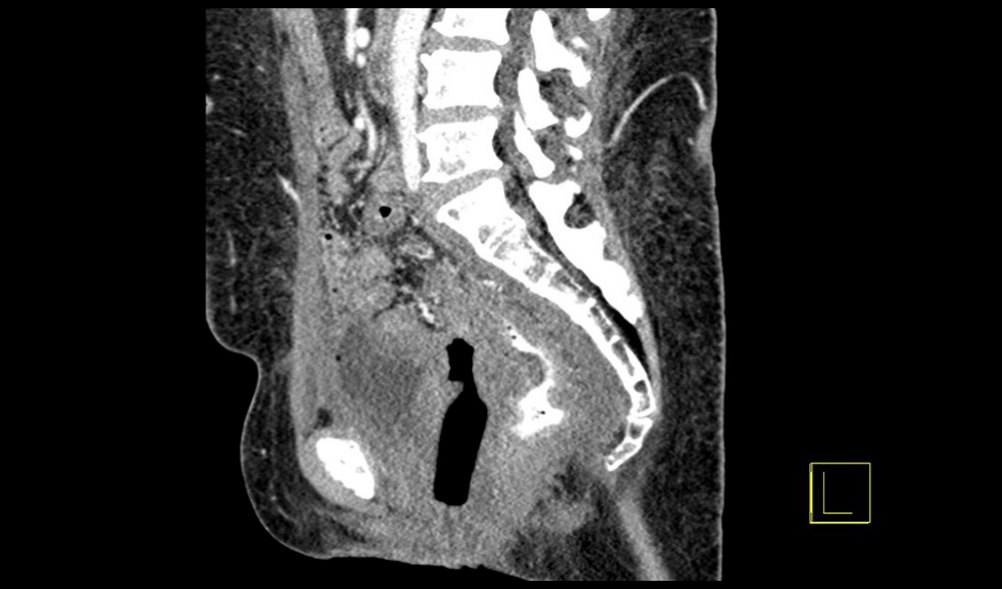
Pre-intravenous contrast CT scan (sagittal reformat) showing the vaginal tampon *in situ* within the vagina for case 1.
